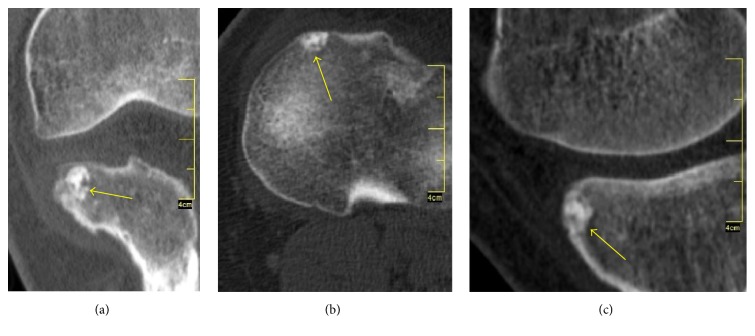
Coronal (a), axial (b), and sagittal (c) CT images showed the perinidal sclerosis with centrally calcified nidus (yellow arrow) consistent with an osteoid osteoma.
